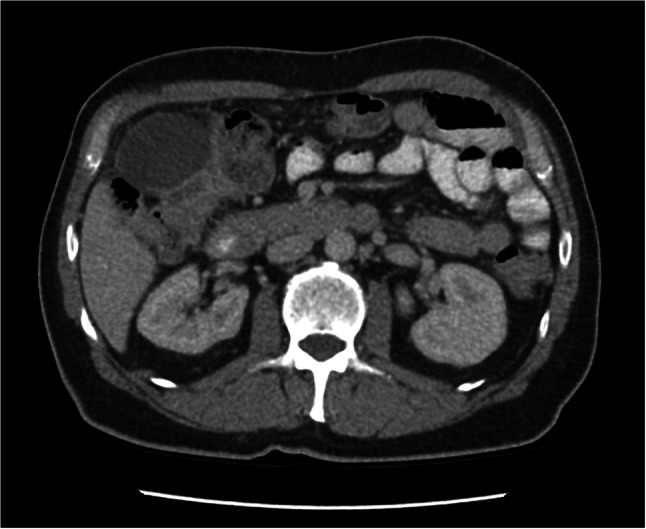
Axial view of abdominal CT showing mass at hepatic flexure
